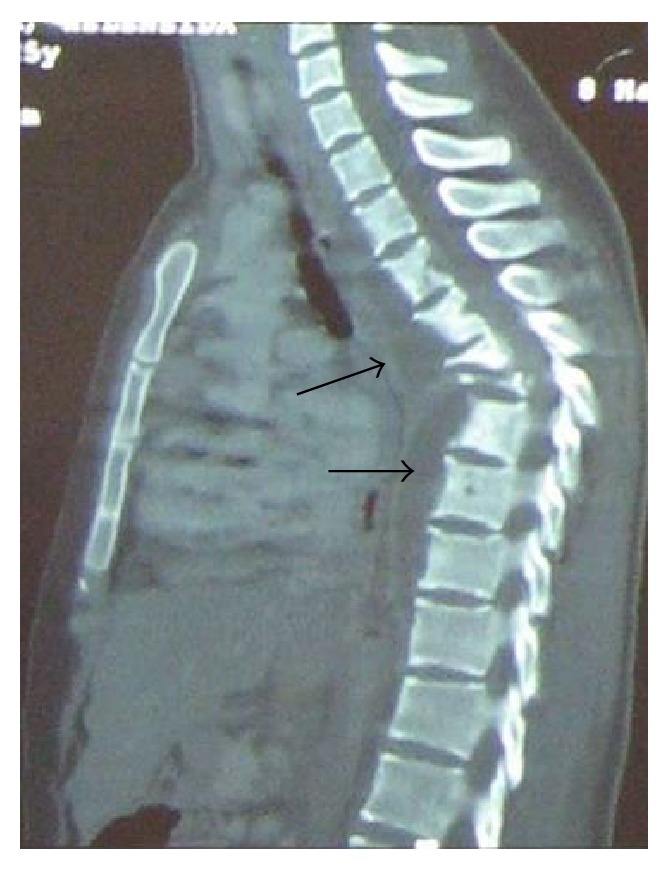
CT showing osteolytic lesions involving several vertebral bodies with severe kyphotic deformity, due to the collapse of the anterior spinal elements.
